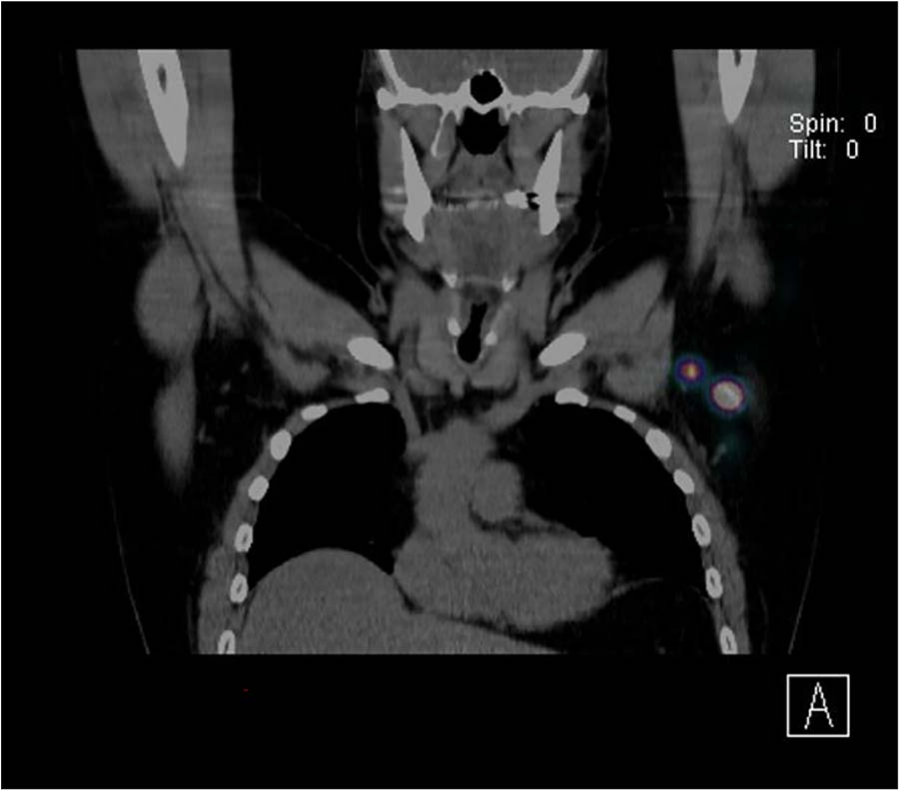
CT SPECT image identifying two SLNs in the left axilla.
